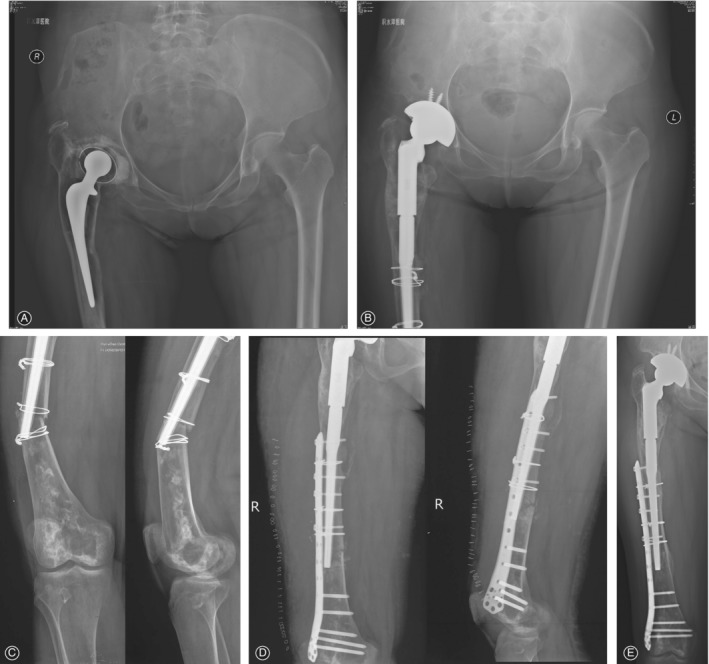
(A) Preoperative anteroposterior (AP) radiograph of the right hip in a patient requiring revision because of aseptic loosening. (B) AP radiograph 2 months after revision arthroplasty with a 64‐mm diameter cementless acetabular component and a modular revision stem. (C) The patient experienced a Vancouver B1 periprosthetic femoral fracture 13 months later. (D) The patient was treated with open reduction and internal fixation (ORIF) of the femur. (E) AP radiograph 3.5 years after the ORIF.
